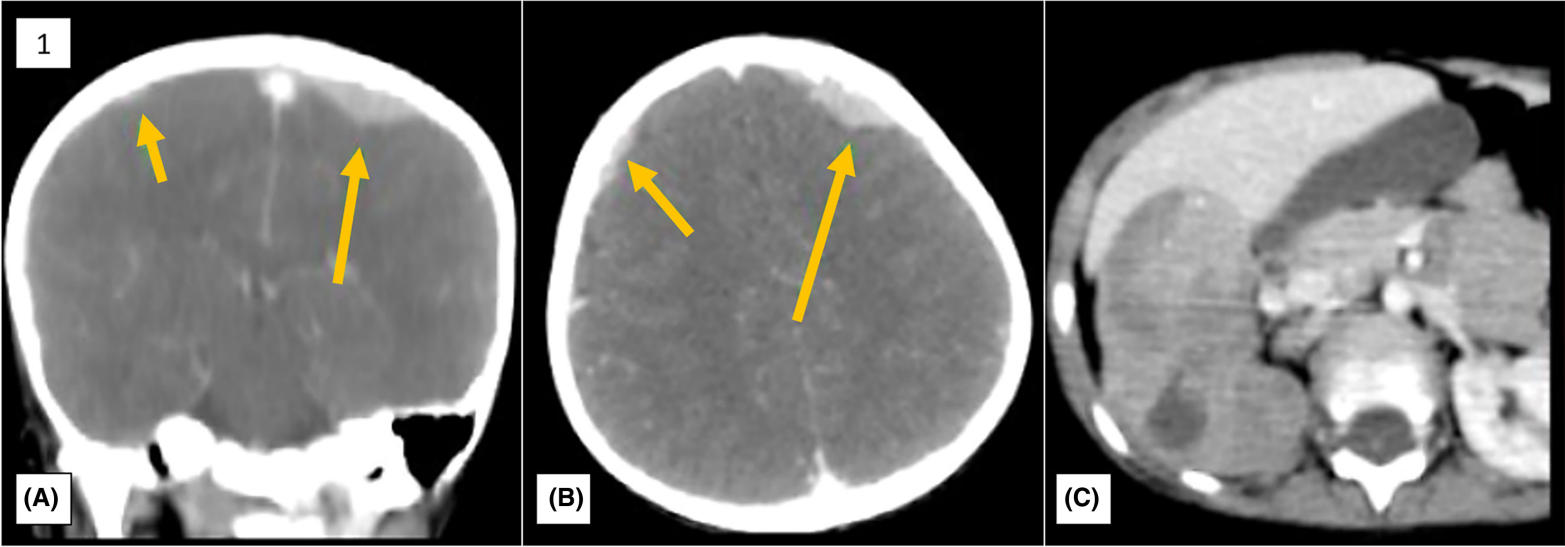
F/18 FDG PET/CT scan from head to mid‐thigh: FDG uptake increased in bilateral frontal and parietal regions and right suprarenal region.
